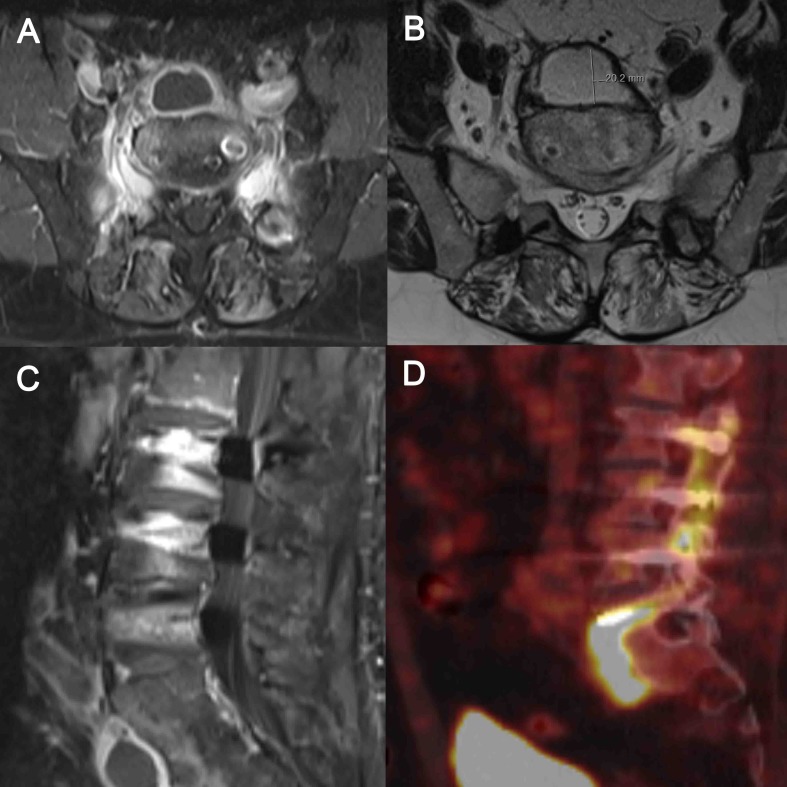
MRI and PET-CT indicated a small abscess anterior (20.2 mm in diameter, B) to segment L5/S1. A, transversal T1 sequence of MRI with contrast medium. B, transversal T2 sequence of MRI with contrast medium. C, sagittal T1 sequence of MRI with contrast medium. D, PET-Scan of the lumbar spine.
